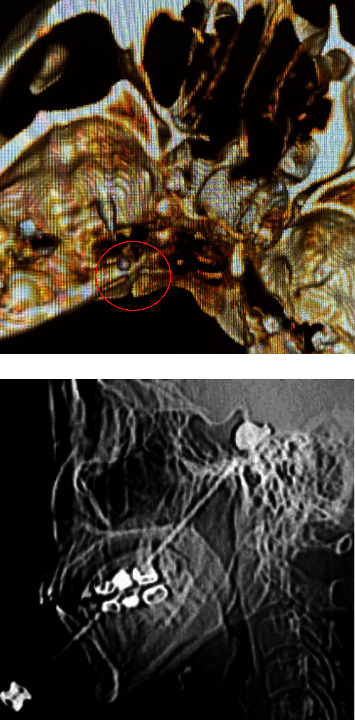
The location of the balloon catheter can be determined by inserting the balloon catheter under CT guidance. After the iohexol was injected, a “pear-shaped image” was displayed on lateral CT imaging, indicating successful surgery.
